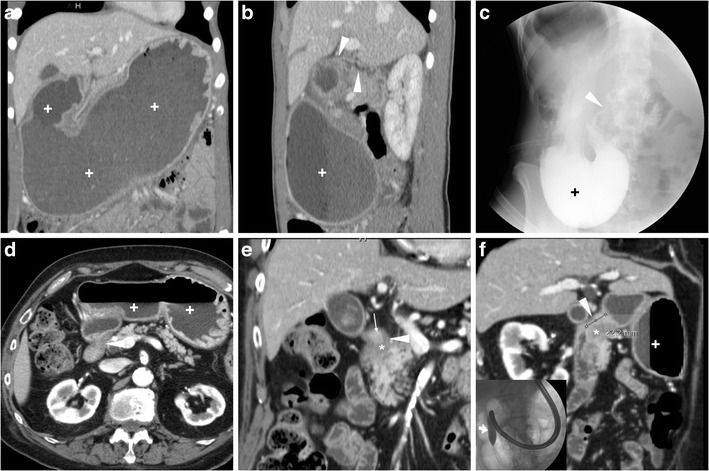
A young 21-year-old female with history of HP infection experienced gastric dilatation, relieved with nasogastric intubation. Despite inability to drink, contrast-enhanced CT (a, b) showed markedly dilated fluid-filled stomach (+), contracted duodenal bulb with 3-cm long stricture (arrowheads in b) without mural thickening, abnormal solid tissue and extrinsic masses. Upper digestive double-contrast fluoroscopy (c) showed dilated stomach with stagnant barium (+) and poorly distensible gastric outlet (arrowhead). Endoscopy confirmed deformed, impassable stricture and two small-sized pyloric ulcers with bioptic finding of severe acute and chronic inflammation. A 88-year-old diabetic male suffered from vomiting, epigastric pain and dark stools. Contrast-enhanced CT (d…f) confirmed radiographic finding of gastric dilatation unrelieved by nasogastric tube, with intraluminal stagnant fluid (+) and depicted a 2-cm pyloric-duodenal stricture (arrowheads in e, f) with subtle submucosal hypoattenuation (*) and mucosal hyperenhancement (thin arrow in e) but no signs of mass-forming or infiltrative disease. Biopsies confirmed post-inflammatory stricture, which was treated by endoscopic dilatation
